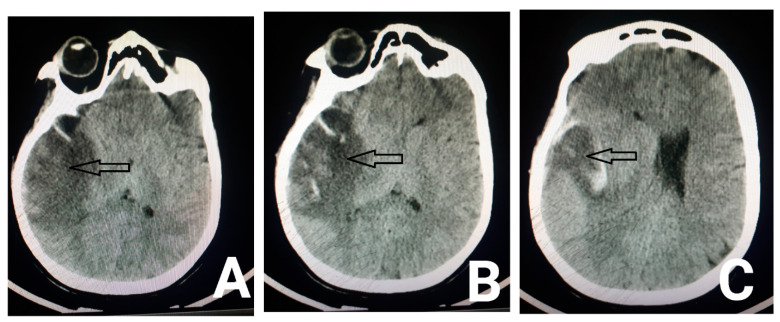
Axial brain CT scan shows hypodensity in the right temporal lobe (arrows in Figure A,B) in the drainage territory of the vein of Labbe, indicating venous infarct with parenchymal hemorrhage (arrow in figure (C)).
